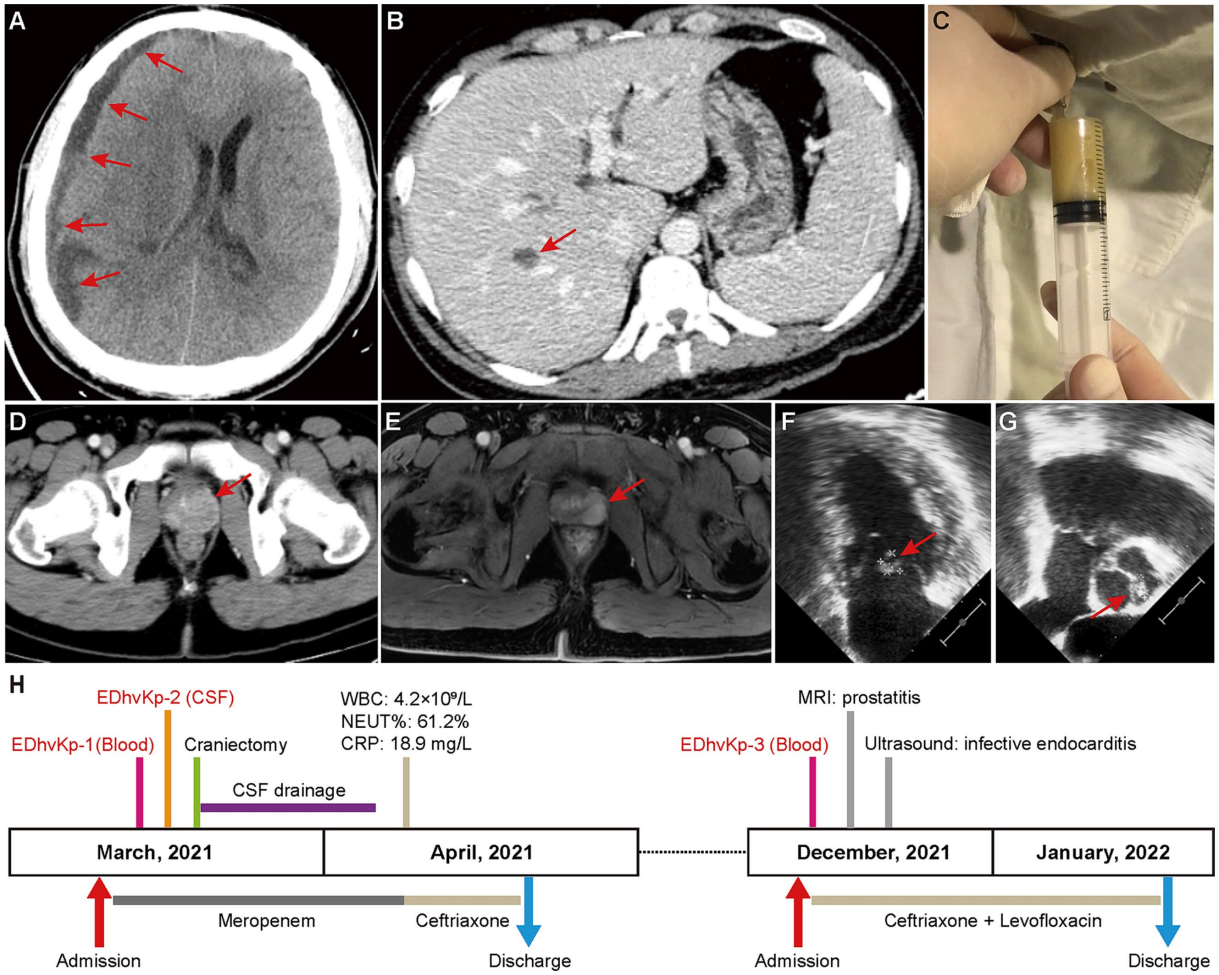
Clinical image findings and treatment process of this case. (A) CT-scan showed a subdural effusion compressing brain tissue. (B) CT-scan showed a liver abscess in right lobe, which disappeared in a subsequent CT scan after antibacterial treatment, supporting the diagnosis of a liver abscess. (C) Purulent CSF was drained by lumbar puncture. (D,E) Contrast-enhanced CT-scan and magnetic resonance imaging showed prostatitis. (F,G) Cardiac ultrasound suggested excrescences on the bicuspid valve (9.3 × 8.4 mm) and aortic valve (8.8 × 5.3 mm). (H) Clinical characteristics and treatment process of the patient.
